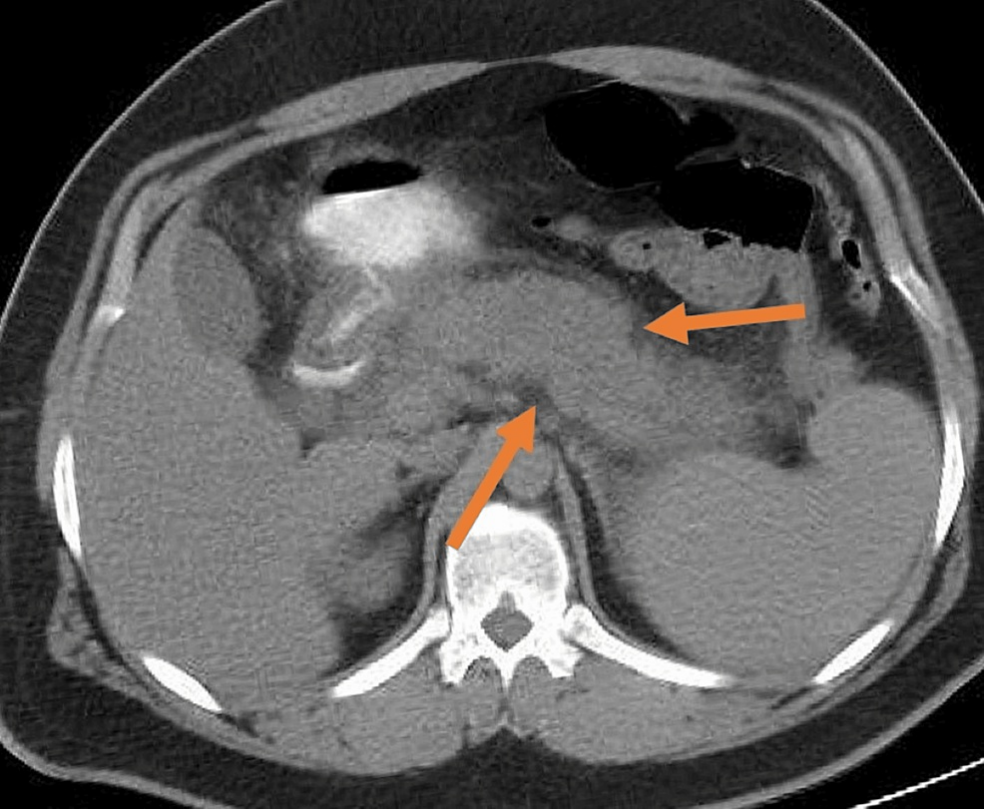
CT abdomen revealing diffuse inflammation of the pancreas with ill-defined borders and fat stranding CT: computed tomography
